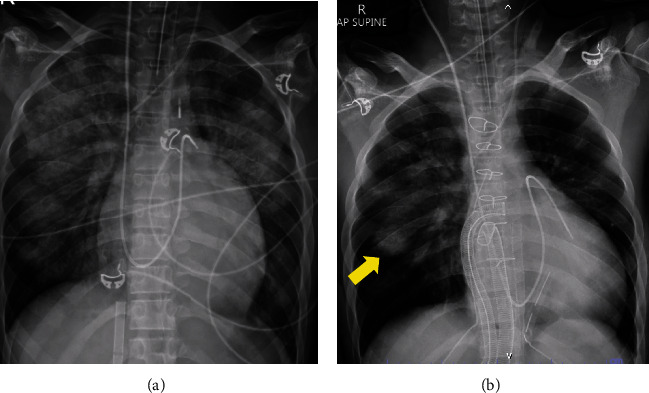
Comparison of chest X-ray findings on the 1^st^ day and 9^th^ day of admission. (a, b) On the 9^th^ day of admission, after adequate decongestion, the follow-up chest X-ray revealed persistent pulmonary infiltration right middle lung zone (yellow arrow).
